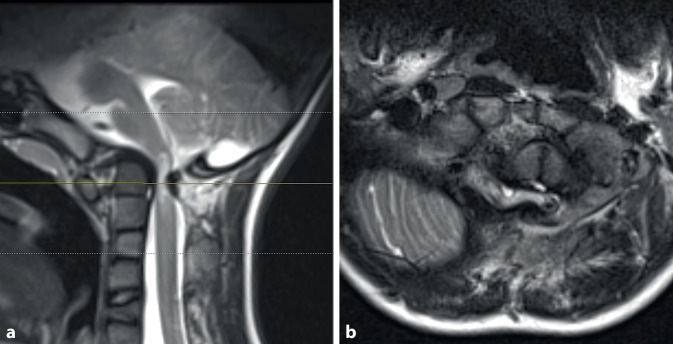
Magnetic resonance images in sagittal (a) and axial (b) planes showing the severity of cervical stenosis with myelopathy at C1 level
